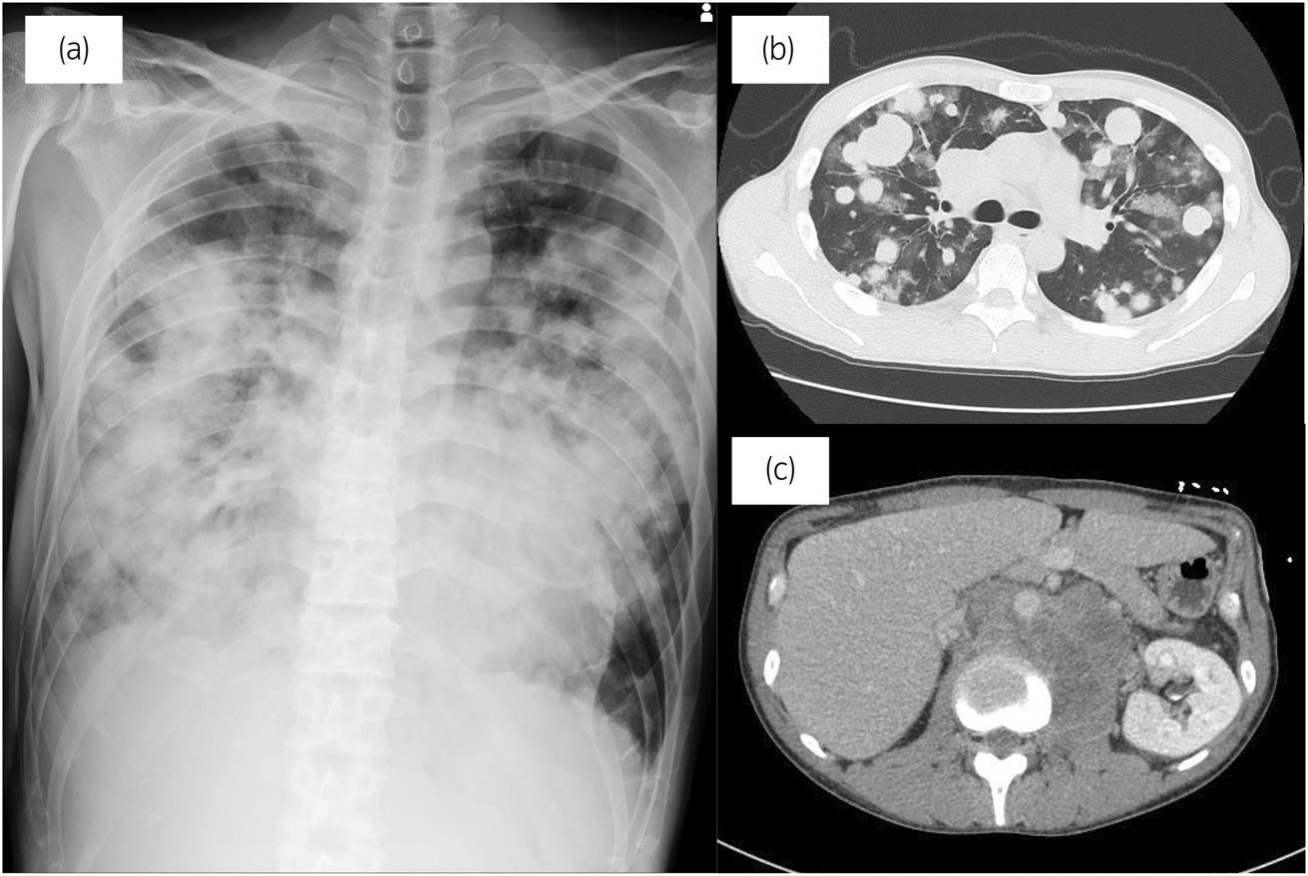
Image before treatment. (a) Chest X‐ray showed extensive infiltrative shadows in the lung fields. (b) Chest CT scan showed multiple nodular shadows with ground‐glass opacities suggestive of hemorrhage in the lung. (c) Abdominal contrast‐enhanced CT scan showed enlarged retroperitoneal lymph nodes.
